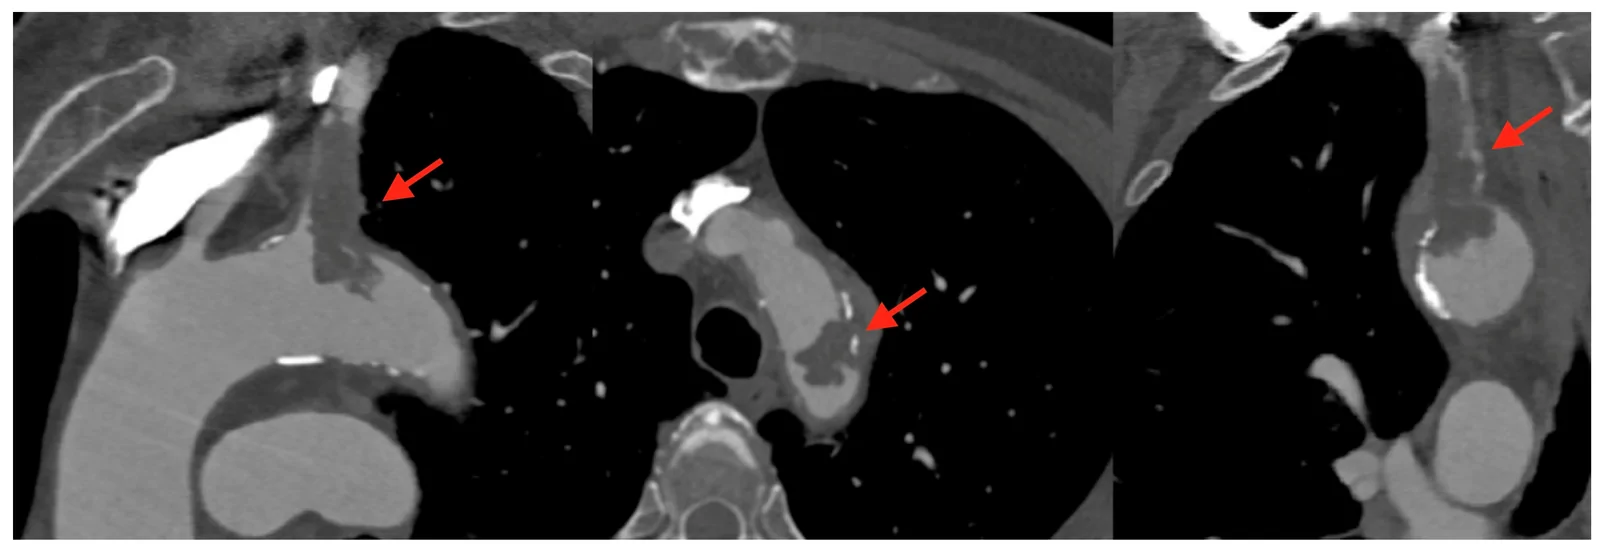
Computed tomography angiography at presentation. Representative multiplanar reconstruction showing the sub-occlusive intraluminal filling defect extending from the left subclavian artery (arrow) with extension towards the aortic arch, initially interpreted as thrombus.
